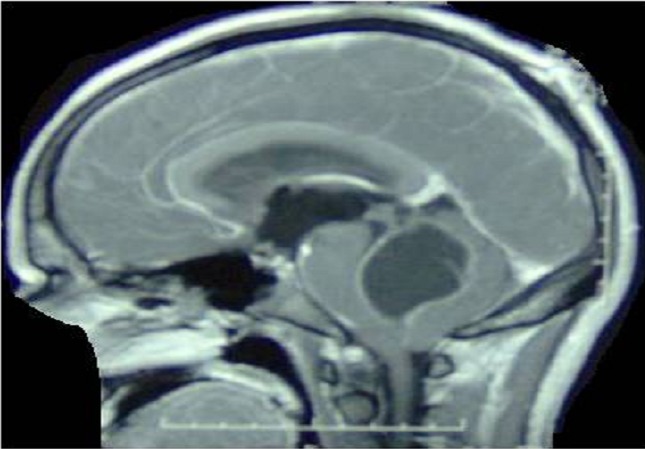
Brain MRI T1 sagittal sequence after Gadolinium injection showing homogeneous wall enhancement
